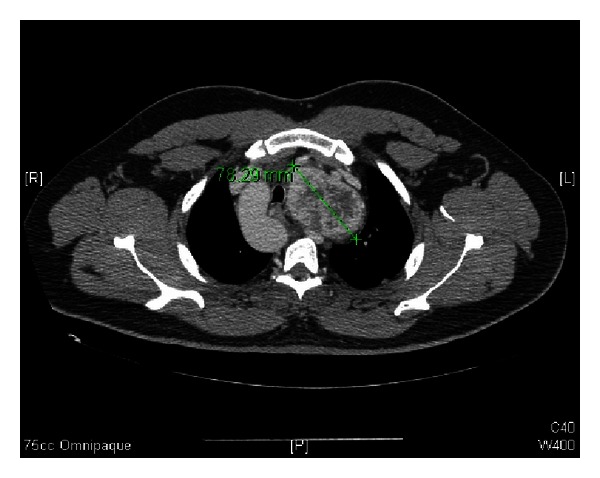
Axial CT scan with contrast at T4, showing retrosternal extension of the left thyroid lobe, with maximal diameter of 78 mm.
